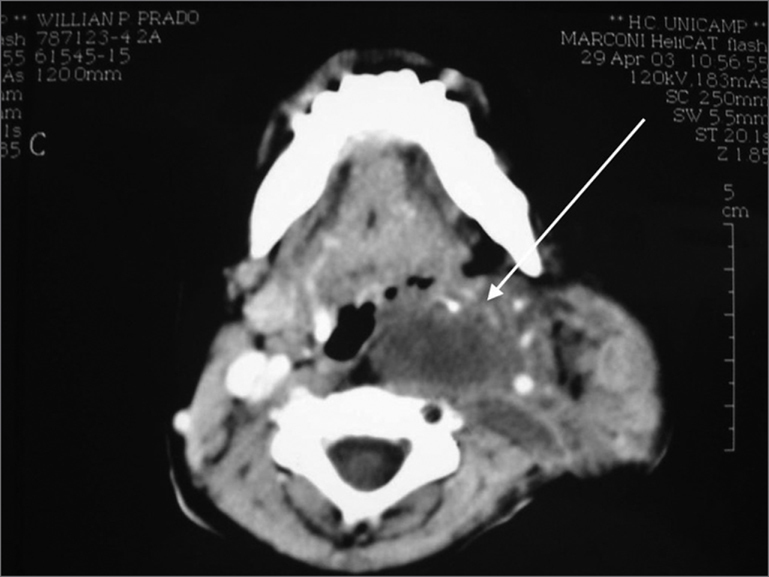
Cervical Neurofibromatosis - see arrow for tumor in neck CT scan axial view.
